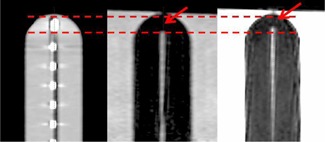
A paracoronal view of the CT (left), T1W (middle), and T2W (right) images of a 30 mm standard vaginal applicator with the appropriate CT or MR marker. In contrast to the CT, the MR markers for both the T1W and T2W images were displaced from the tip of the applicator, suggesting the markers were compromised.
